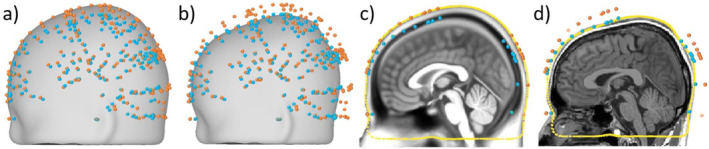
TPS warping of the MNI152 template MRI with 150 scalp digitization points (cyan color) from a 35‐year‐old adult subject: (a) A selection of control points (orange color) on the template scalp (gray color) corresponding to each digitization point; (b) warped scalp surface (gray color) after applying the TPS‐transform; (c) template scalp surface (yellow dashed line) and control‐point pairs overlaid on the template MRI; and (d) warped scalp surface (yellow dashed line) and control‐point pairs overlaid on the real MRI of the subject.
